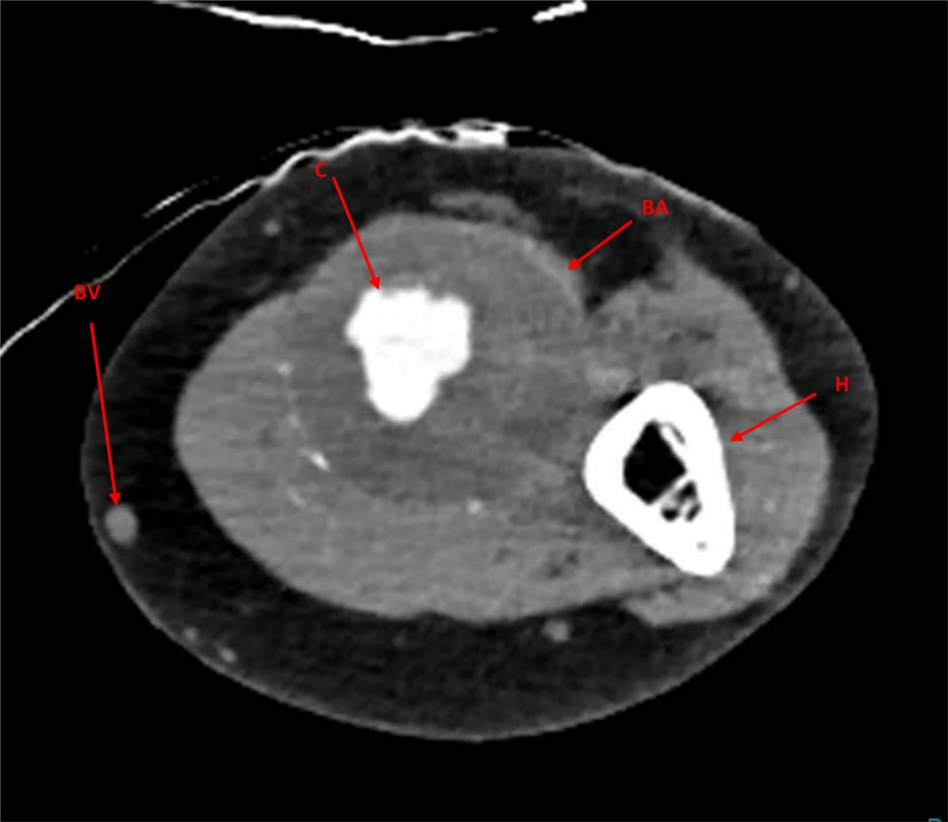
CTA imaging of brachial artery aneurysm of the left arm; BA, brachial aneurysm; BV, basilic vein; C, contrast through aneurysm lumen; H, humerus.
